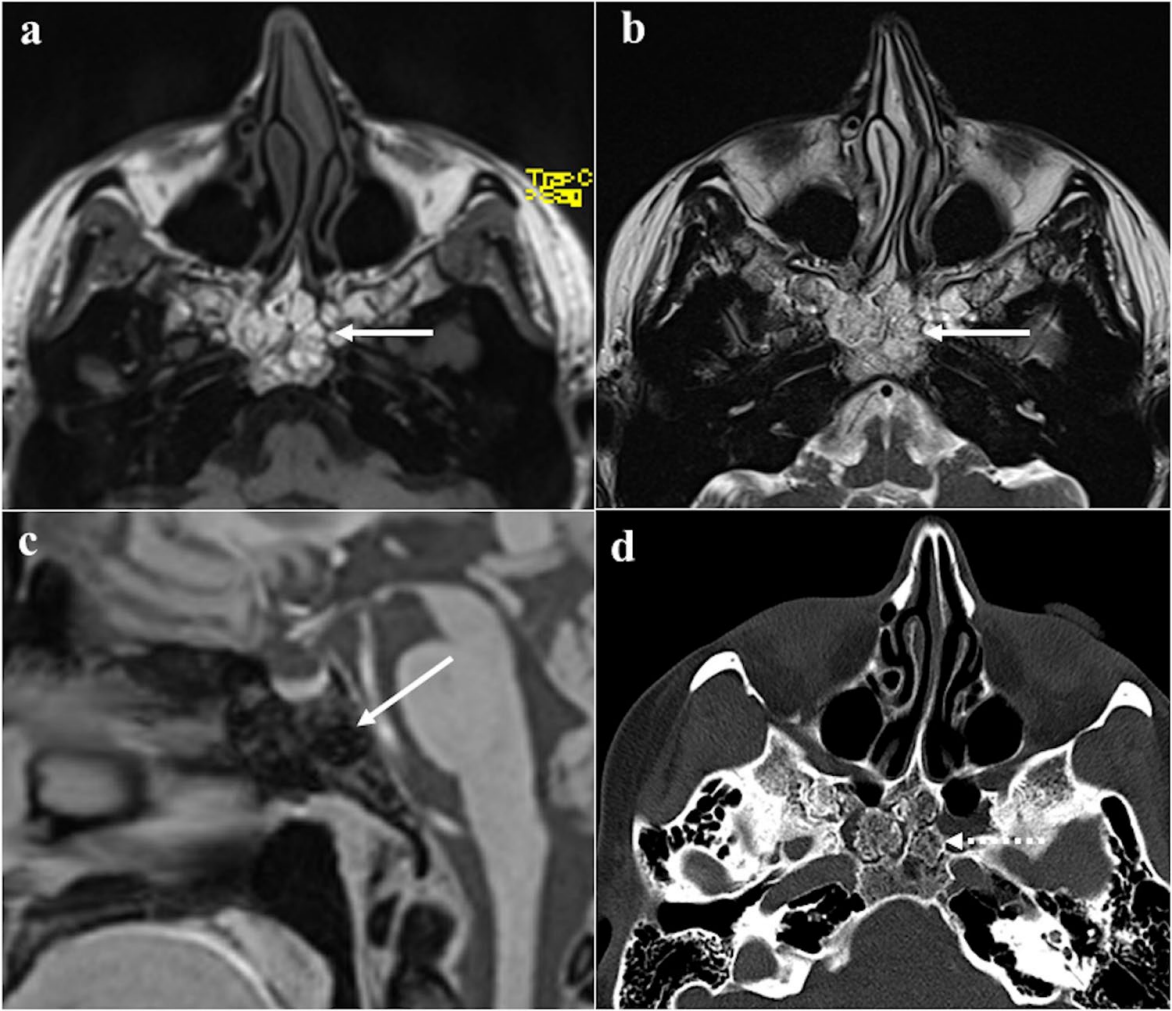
Arrested pneumatisation of the sphenoid sinus as an incidental finding in a 51-year-old female patient with headache. The sphenoid sinus is replaced by a non-expansile solid lesion (white arrows) showing high SI on MRI axial T1W (a) and T2W images (b), and homogeneous low SI on sagittal T1W fat-saturated sequence (c). Axial bone algorithm reconstruction CT image (d) shows a lesion with sclerotic margins, internal curvilinear calcifications, foci of fat, and loss of bone trabeculae (white dotted arrow). Note the absence of a cortical bone breach
